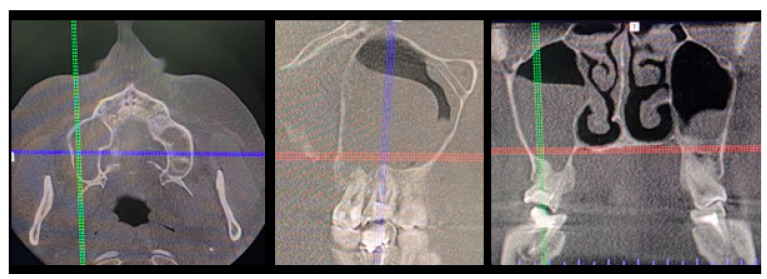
The inappropriate endodontic treatment of the upper second molar led to its inner root resorption, forming of granulation tissue, and the co-incidence of the right maxillary sinus retention cyst. The inflammation and granulation tissues, adjacent teeth, and bone were scheduled for removal. Because of combining wound debridement, cyst, and teeth removal, from the same approach, few different procedures were made. Wound debridement and removal of granulation and inflamed tissues, along with some bone, were scheduled. Because of the presence of oro-antral communication, resulting from the loss of wast bone tissue, the Bihat fat pad was used to close the communication and suture the wound in two layers. The blue, green, and red reference lines are related to CBCT evaluation in axial, sagittal, and coronal views respectively.
